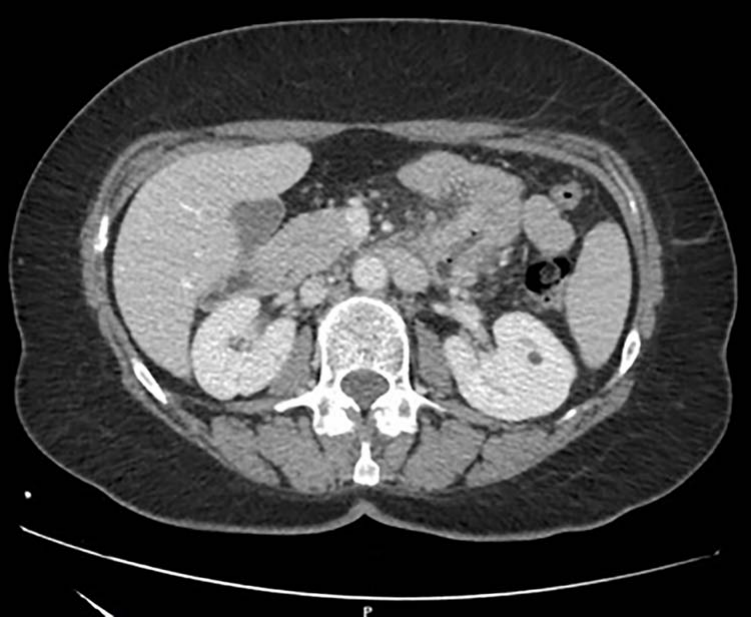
Contrast-enhanced CT scan.
